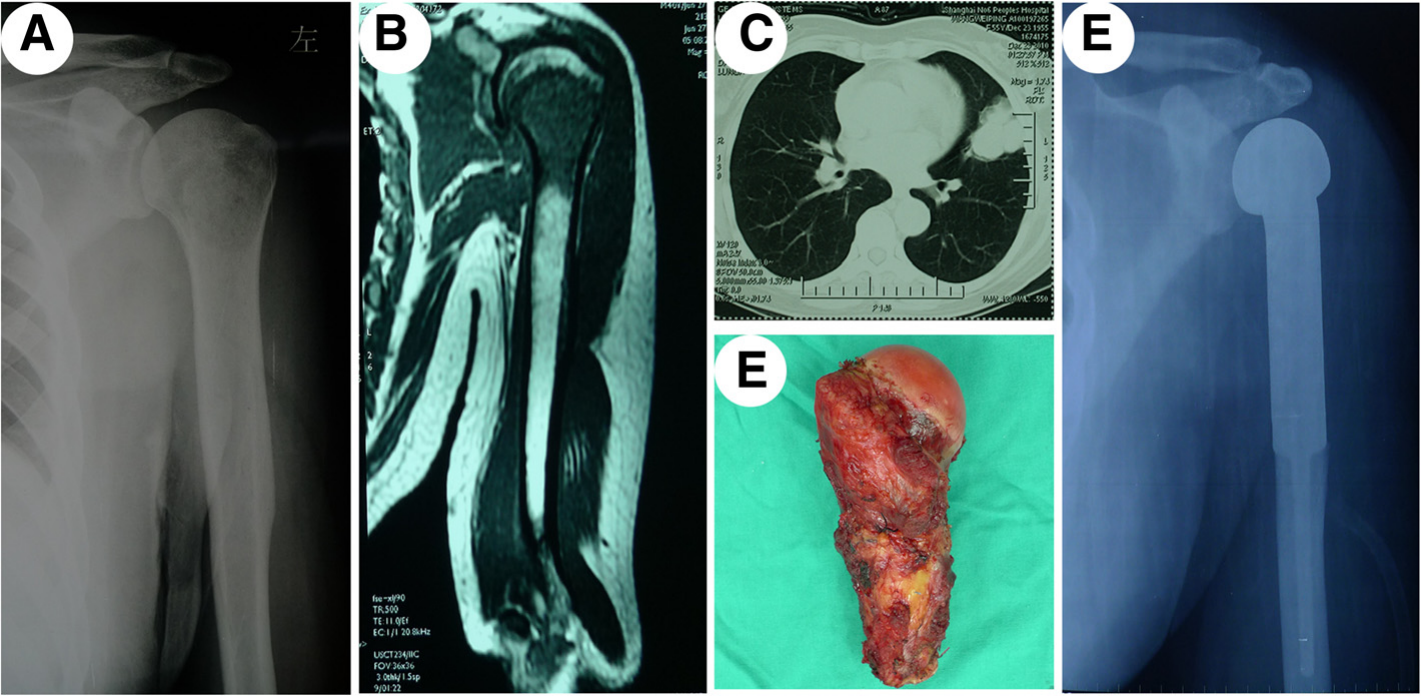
Typical case 2. a X-ray revealed bone destruction at the proximal end of the left humerus; b MRI demonstrated hypointense signal in T1-weighted images; c CT revealed right lung cancer; d postoperative gross observation; e postoperative X-ray
